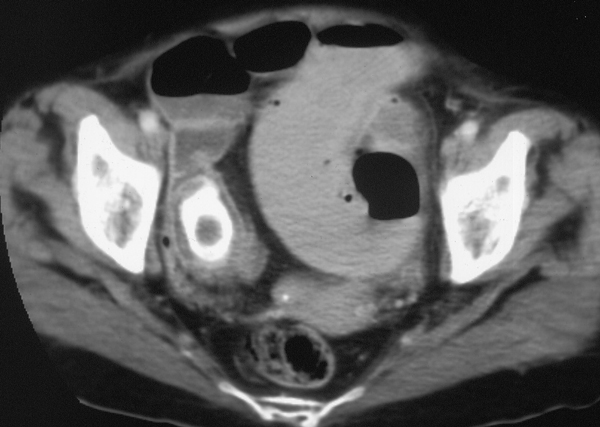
CT scan case 1: The gallstone is clearly detectable within the lumen of ileus.
